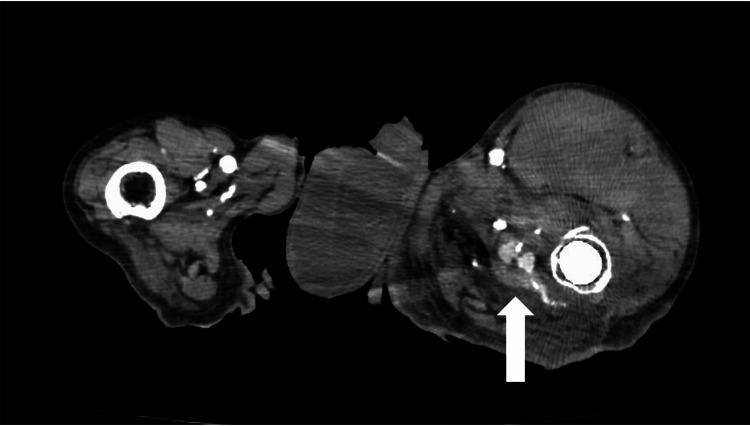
An axial CT scan This shows bleeding within the left thigh (arrow).
